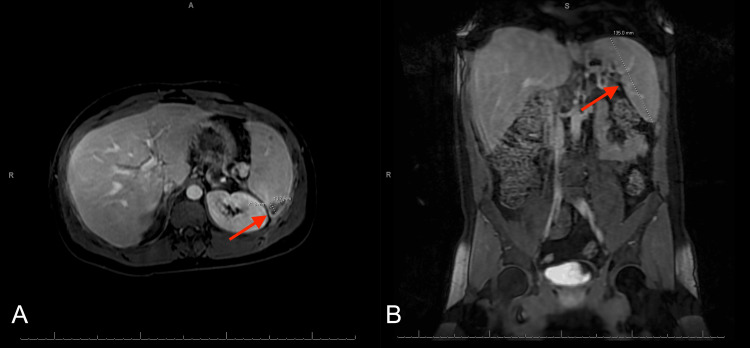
MRI abdomen and pelvis with and without IV contrast (A) Hypointense lesion in the posterior aspect of the spleen measuring approximately 2.1 x 1.3 cm. (B) Splenomegaly measuring 13.5 cm.
